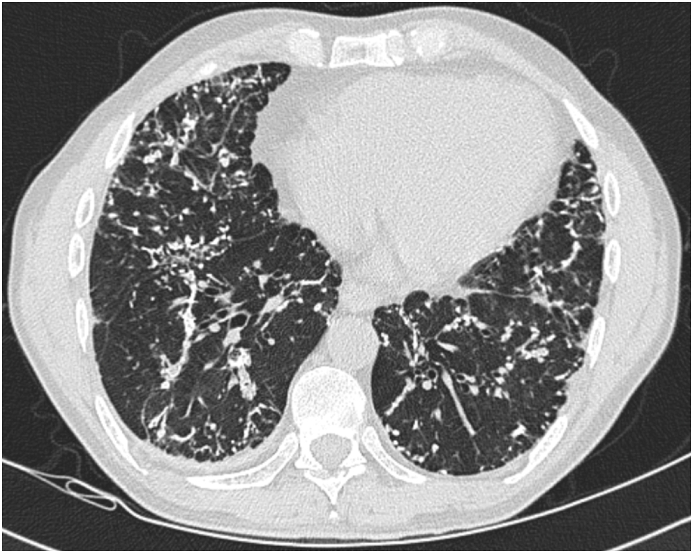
Transverse slice of a high resolution computed tomographic scan of the chest on inspiration with thin slices using lung windowing demonstrating both the bilateral pulmonary opacities, calcifications, and mild bronchiectasis throughout the bilateral lower lobes of the lungs.
